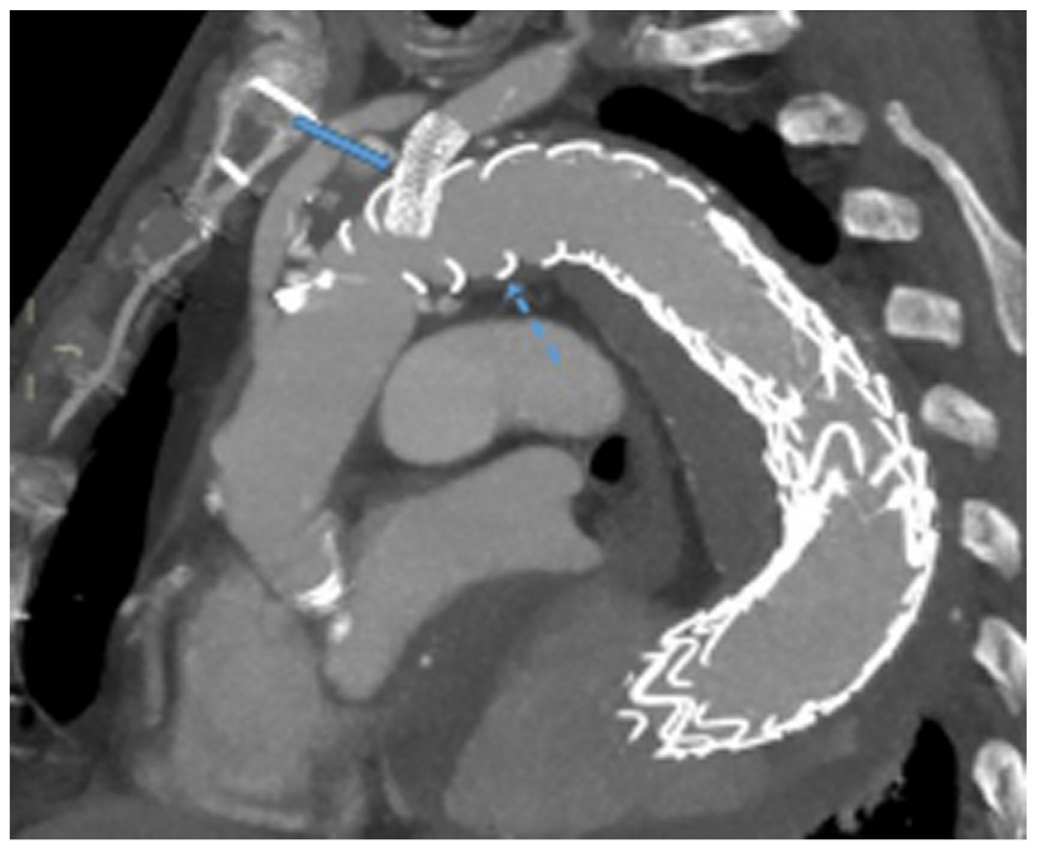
Three weeks after the last procedure, the patient had a follow-up computed tomography angiogram showing a patent left subclavian artery (LSA) stent (*arrow*) and no endoleak. *Dashed arrow* indicates the Thoraflex stent graft.
